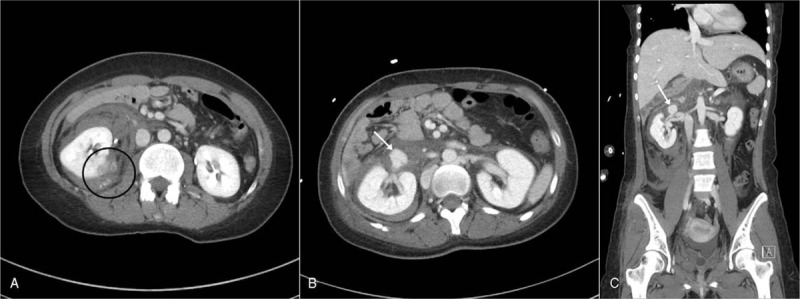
Contrast-enhanced abdominal computed tomography (CT) scan conducted in the emergency department revealed (A) a contrast leakage, which might indicate active bleeding (inner part of the black circle) in the mid-posterior part of the laceration and a hematoma in the perinephric space. Abdominal CT scan showed a pseudoaneurysm (white arrows) of the right renal vein on axial (B) and coronal (C) images.
